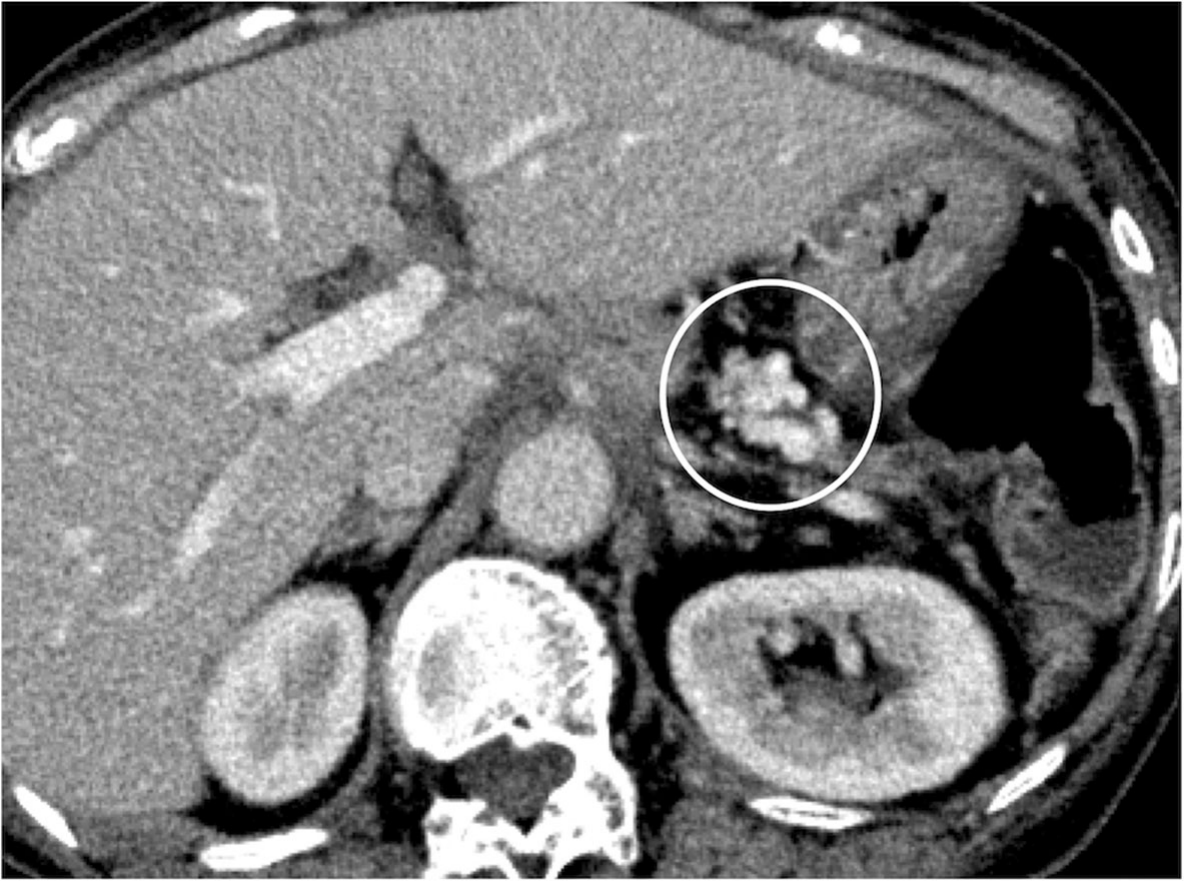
Pancreaticojejunostomy varices remained by follow-up CT at 3 years after first stage PSE
